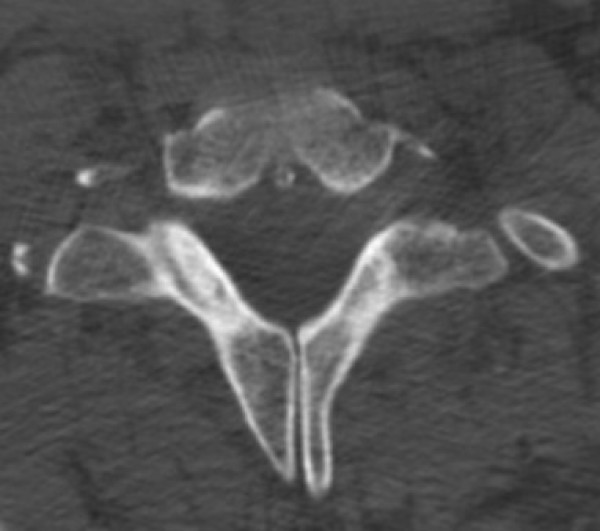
Axial computed tomography scan at the level of T1 vertebra (bone windows). Bifid spinous process of T1 vertebral body is present.
